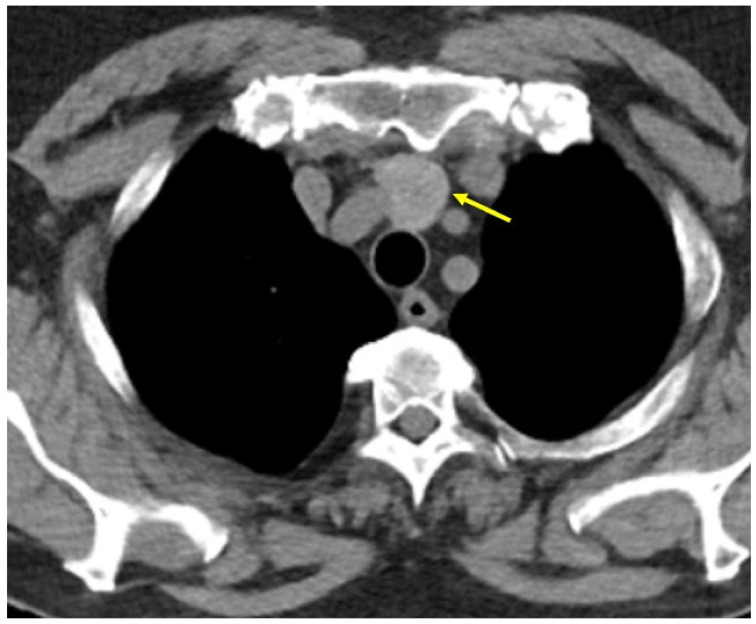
ETTM first suspicion (yellow arrow) amid contrast CT evaluation: a nodular, hyper-dense mass, with inhomogeneous iodophilia, of 2 cm (transverse diameter) by 2.5 cm (antero-posterior diameter) by 3.1 cm (cranio-caudal diameter), located on the median line of the upper mediastinum, posterior to the manubrium, anterior to the trachea, inferior to the thyroid gland and superior to the aortic arch (axial plane).
